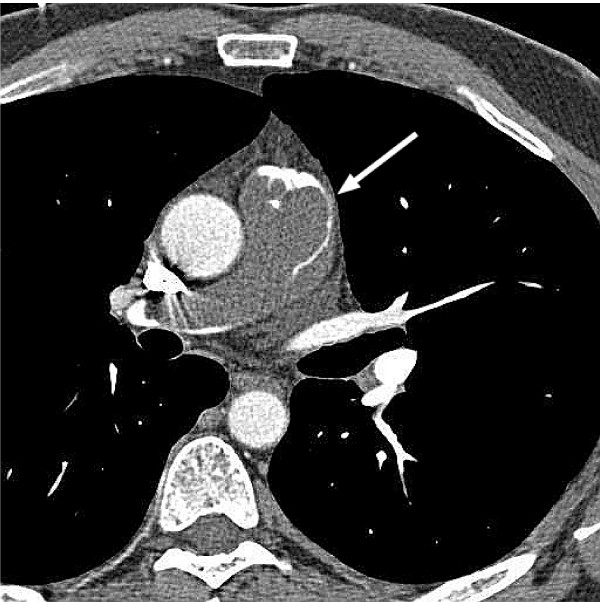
CT scan showing extensive involvement of the main pulmonary artery and into the right trunk.
